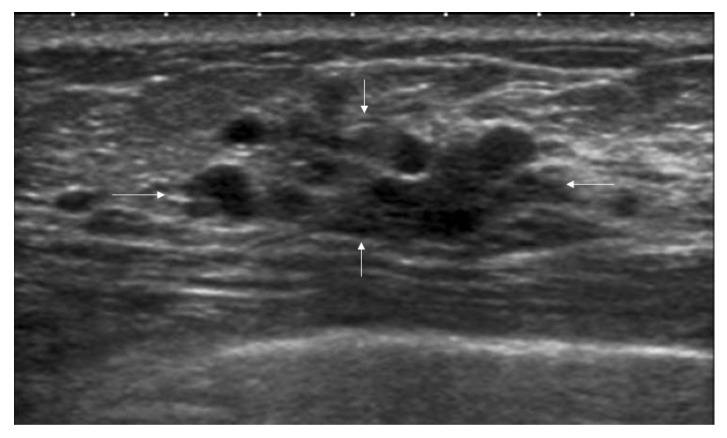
Ultrasound features of non-mass breast lesions of Type IV. A case of ductal papilloma. Ultrasound shows multiple small cysts interspersed with hypoechoic areas (indicated by the white arrows).
